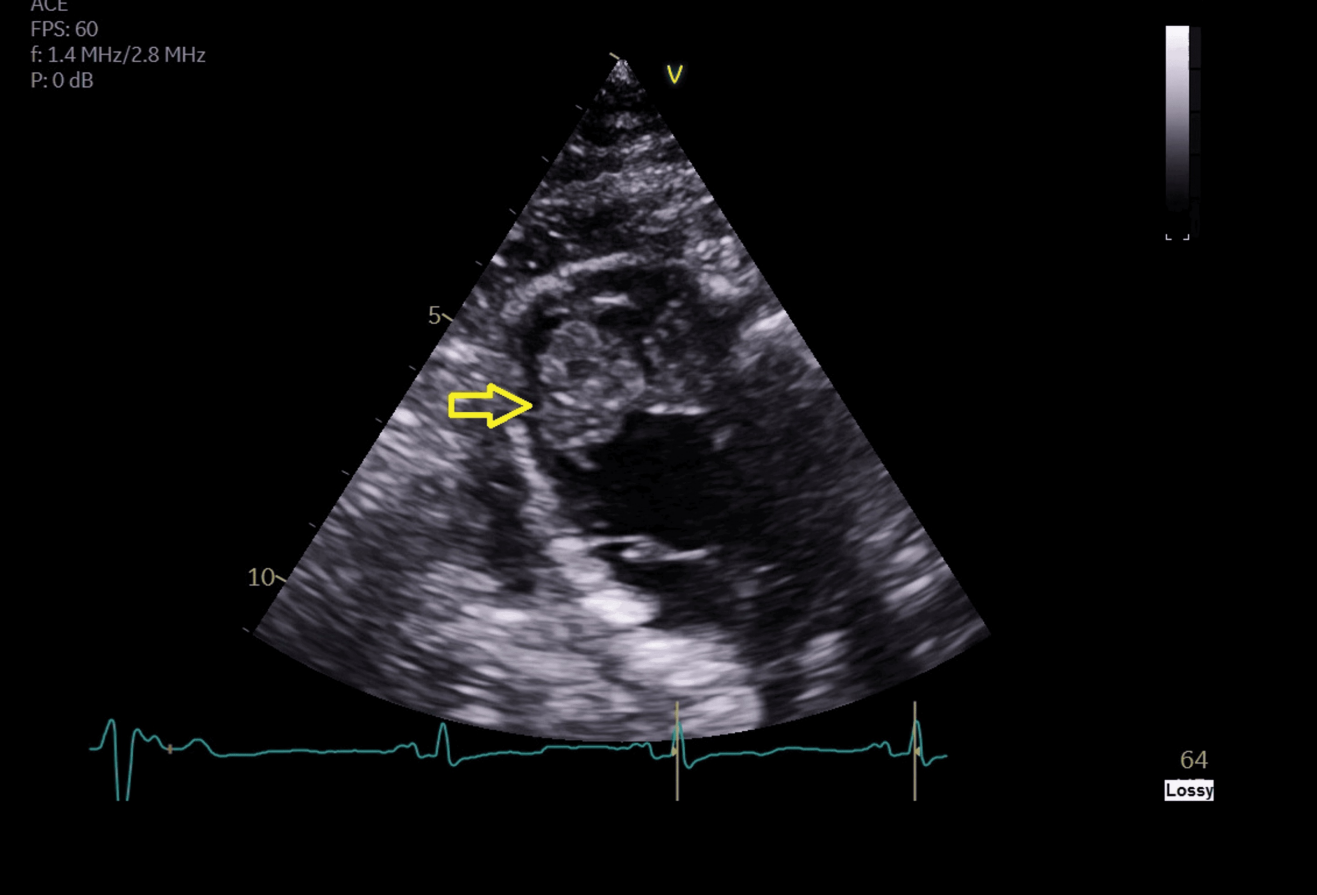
Echocardiogram showing a mural thrombus in the left ventricle Transthoracic echocardiogram (parasternal short-axis view at the mid-ventricular level) showed a mural thrombus (yellow arrow) attached to the left ventricular endocardium. The thrombus appears echogenic and distinct from the adjacent myocardium.
